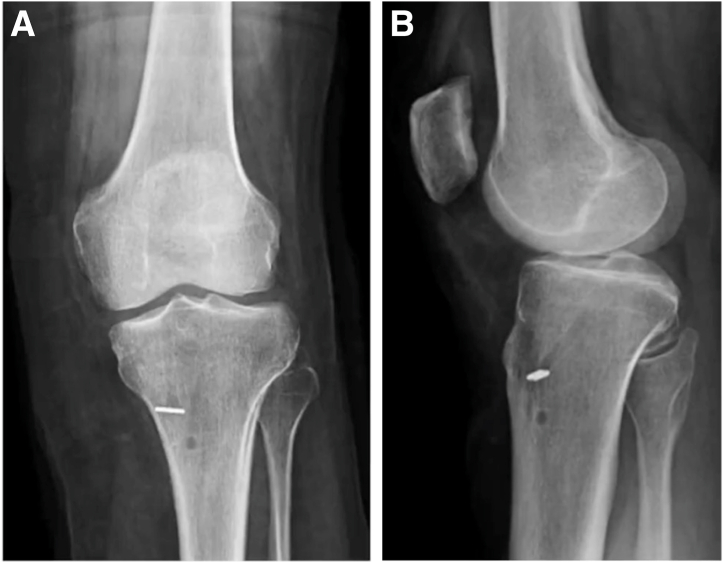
(A, B) Postoperative radiographs left knee after medial meniscal root repair with centralization.
